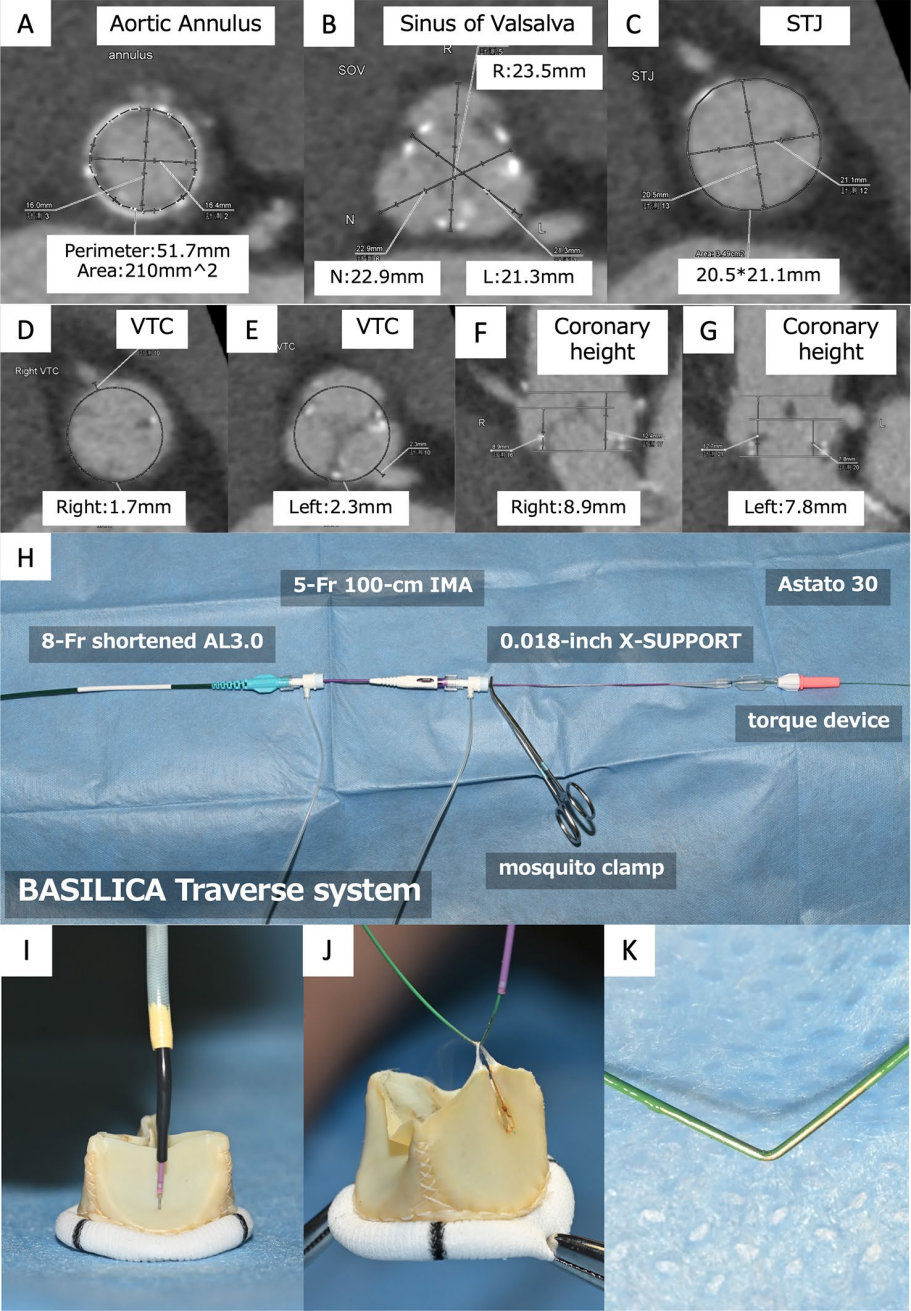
Pre-procedural measurement and pictures of in vitro experiment. a–g Pre-procedural measurements of multidetector computed tomography revealed a small bioprosthetic aortic annulus and tiny virtual transcatheter heart valve to coronary ostial distances. h–k Pictures taken in an in vitro experiment. h BASILICA leaflet traversal system. Catheters and a wire are fixed with a mosquito clamp and a torque device. i Distal configuration of the traversal system shows only the tip of the wire exposed to the leaflet. j Electrified wire was lacerating the leaflet accompanied by smoke and char formation. k Mid-portion of the Cruise wire was kinked and denuded only the inside surface with a scalpel blade
